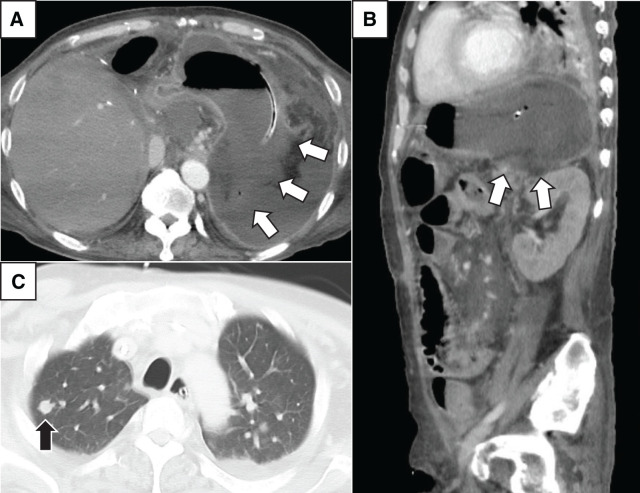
Contrast-enhanced CT findings. (A and B) White arrows show poor contrast enhancement of the gastric mucosa. (C) Black arrow indicates a pulmonary nodule, suggesting disseminated mucormycosis.
